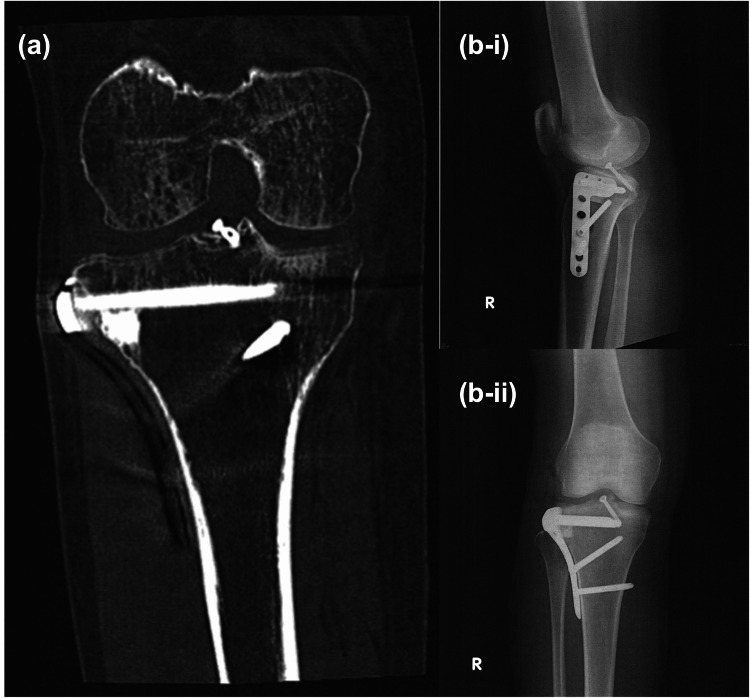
Radiological findings of the tibial plateau fracture after surgery (a) The coronal plane of computed tomography (CT) image after surgery. (b-i) Lateral X-ray findings. (b-ii) Frontal X-ray findings.
